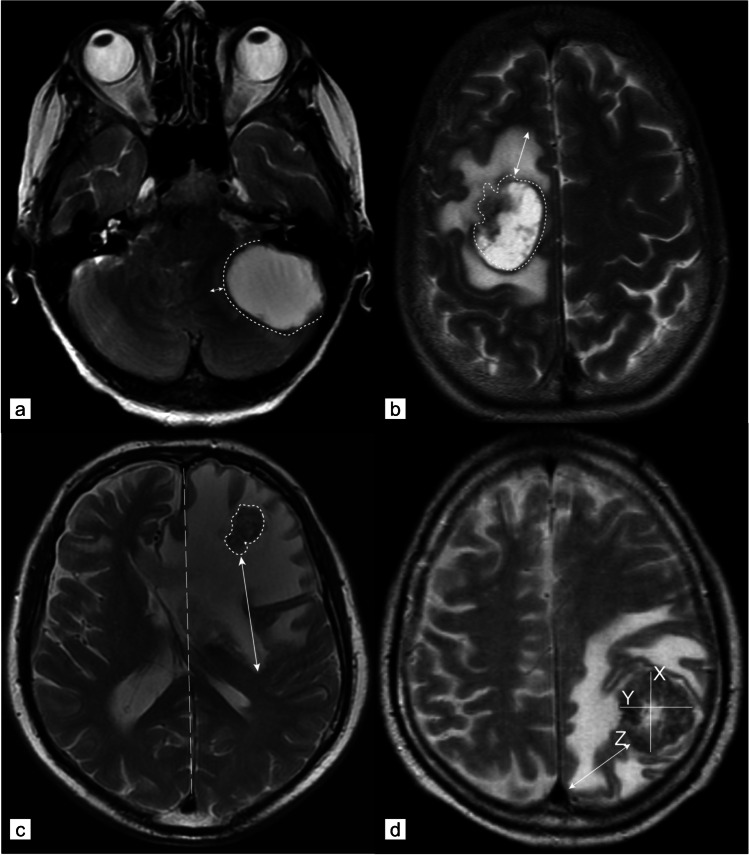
Oedema scoring. Overview of oedema scoring according to Spanberger et al. [9] and Tung et al. [19]. a T2-weighted MRI of a left-sided infratentorial brain metastasis with a very small peritumoural oedema (arrowed line) according to Spanberger grade 1 (maximal width 1 cm). b T2-weighted MRI of a right frontal metastasis with a moderate peritumoural oedema rim (arrowed line) according to Spanberger grade 2 (maximal width > 1 cm, but not crossing the midline of the brain). c T2-weighted MRI of a left frontal metastasis with a large peritumoural oedema (arrowed line) according to Spanberger grade 3 (maximal width > 1 cm rim and crossing the midline of the brain). d T2-weighted MRI with a left parietal metastasis. Example of measurements: (X) maximal orthogonal diameter, (Y) maximal horizontal diameter, (Z) maximal peritumoural oedema expansion measured from the margin of the metastasis. Peritumoural oedema ratio according to Tung et al. [19] is calculated as follows: ‘peritumoural oedema’ / (‘orthogonal diameter’ + ‘horizontal diameter’) / 2
